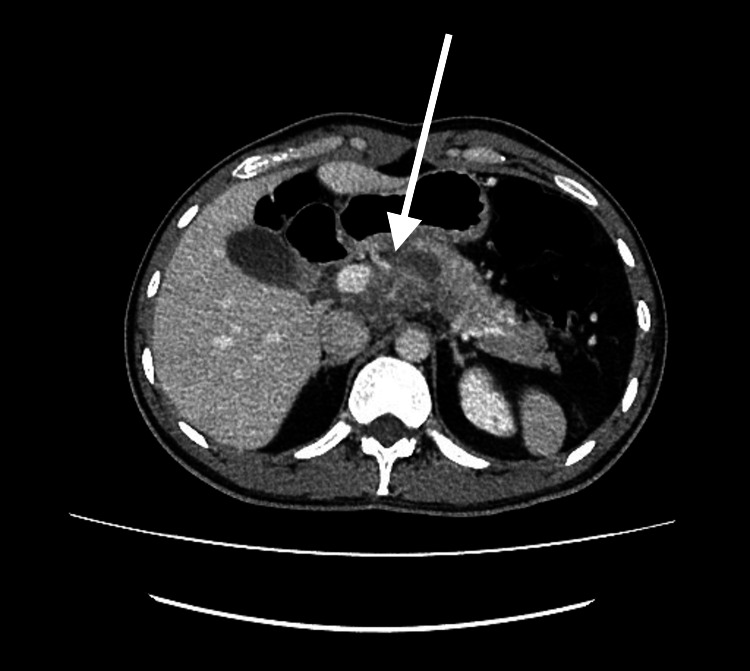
CT thorax abdomen and pelvis with contrast Contrast-enhanced CT showing a multicystic lesion in the pancreatic head/neck region encasing the coeliac axis, with necrotic lymphadenopathy.
